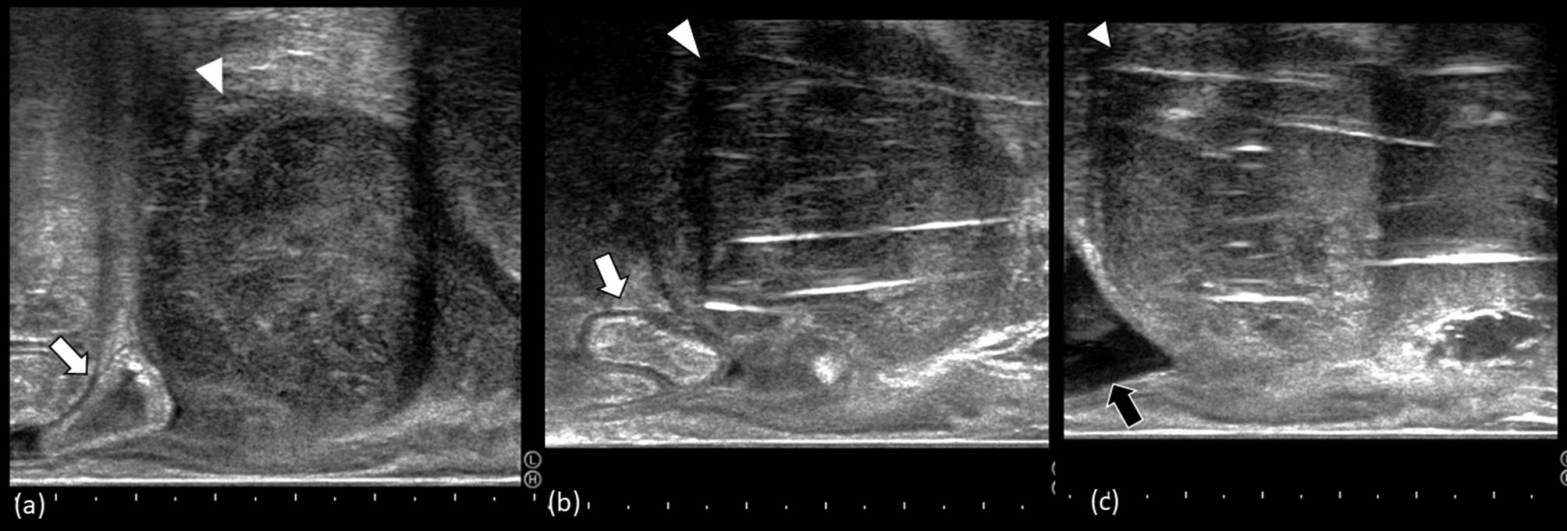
Trans-rectal ultrasonography sagittal view of the recurrent tumor. (a) shows
